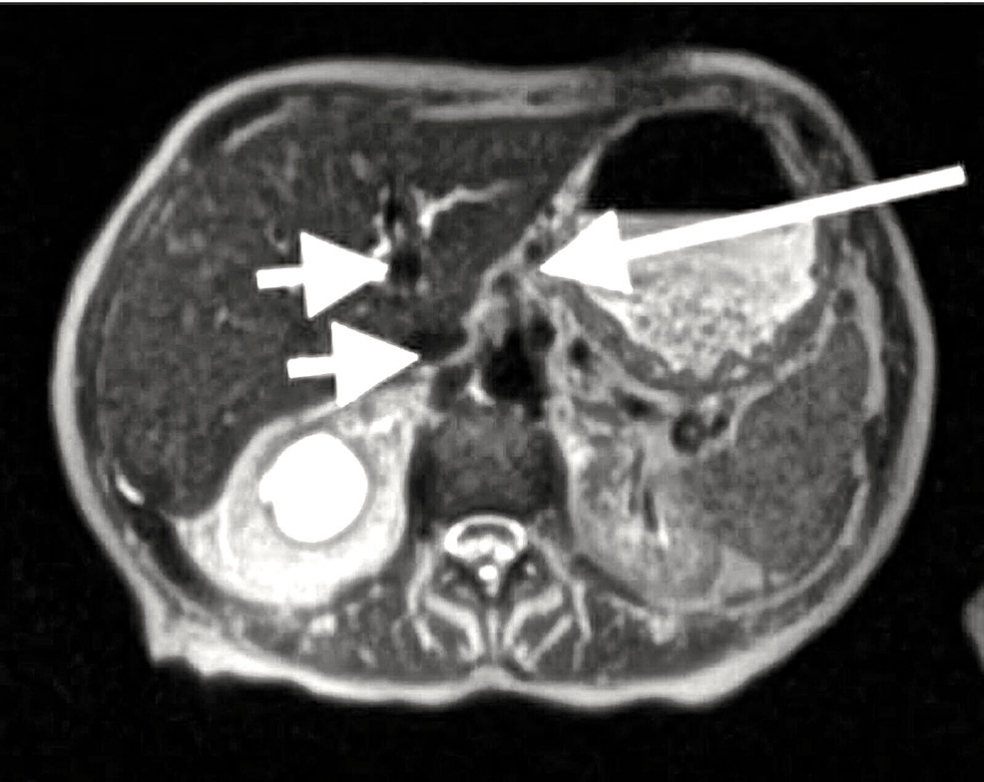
MRI and MRCP abdomen without contrast showing distended stomach to the level of the mid descending segment of the duodenum, at which level there is diffuse mucosal wall thickening (white arrow). There is also intra and extrahepatic biliary ductal dilatation and prominence of the common bile duct to the level of the ampulla (arrowhead). No extraluminal mass is identified. MRI: magnetic resonance imaging; MRCP: magnetic resonance cholangiopancreatography.
